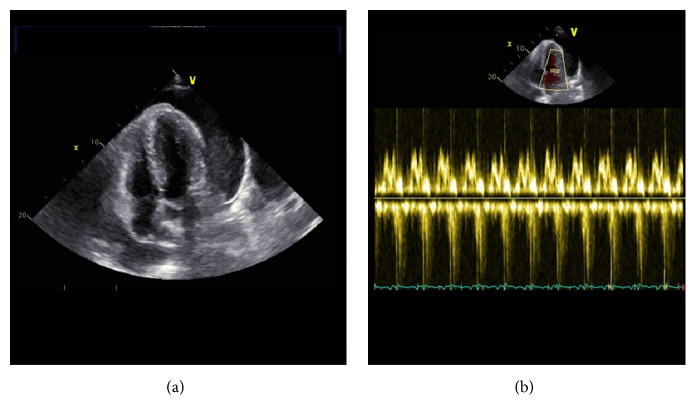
Echocardiogram shows significant pericardial effusion (a) without significant impact on right ventricular function proved by normal mitral inflow (b).
